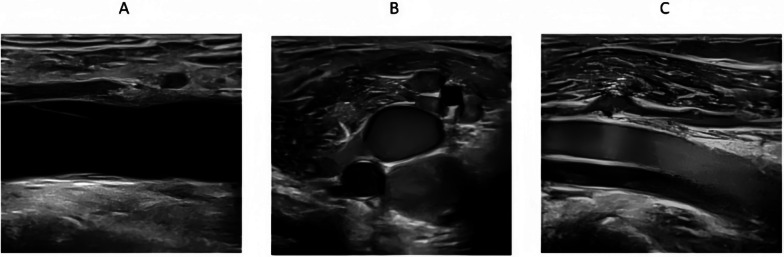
Representative ultrasound images of vascular sections with and without the “blizzard” sign. A Long-axis view of a lower limb vein without the “blizzard” sign. B Short-axis view showing hyperechoic swirling echoes consistent with the “blizzard” sign. C Long-axis view with visible “blizzard” sign indicating red blood cell aggregation due to venous stasis
